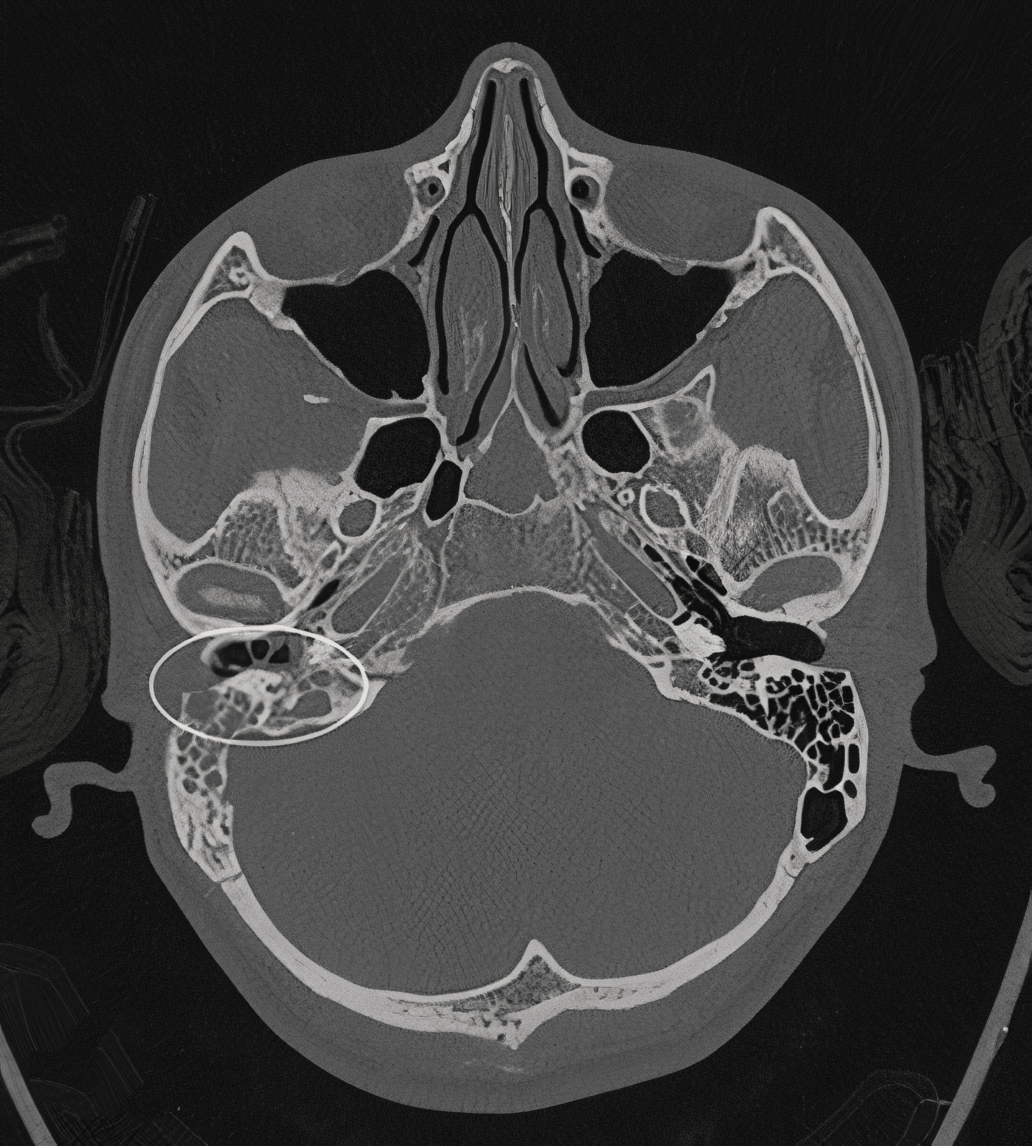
HRCT temporal (bone window, axial view) A right diploid mastoid with a soft-tissue-filled mastoid in the middle ear and external ear canal (circle). The left mastoid is clear. HRCT: high-resolution computed tomography
